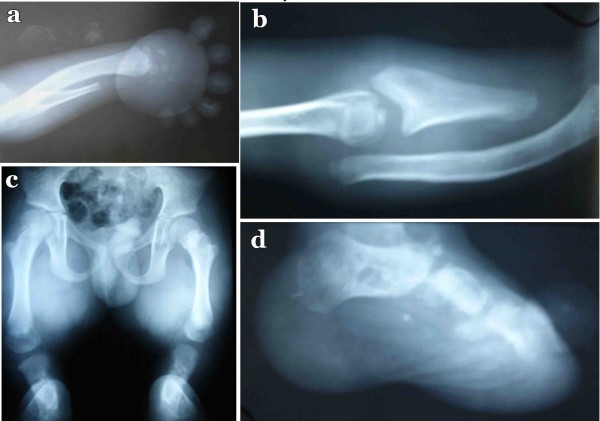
Radiographs of the 10 year's old affected boy (IV-4) with Grebe type chondrodysplasia. Radiographs of hand (a), arm (b), pelvis (c) and foot (d).
